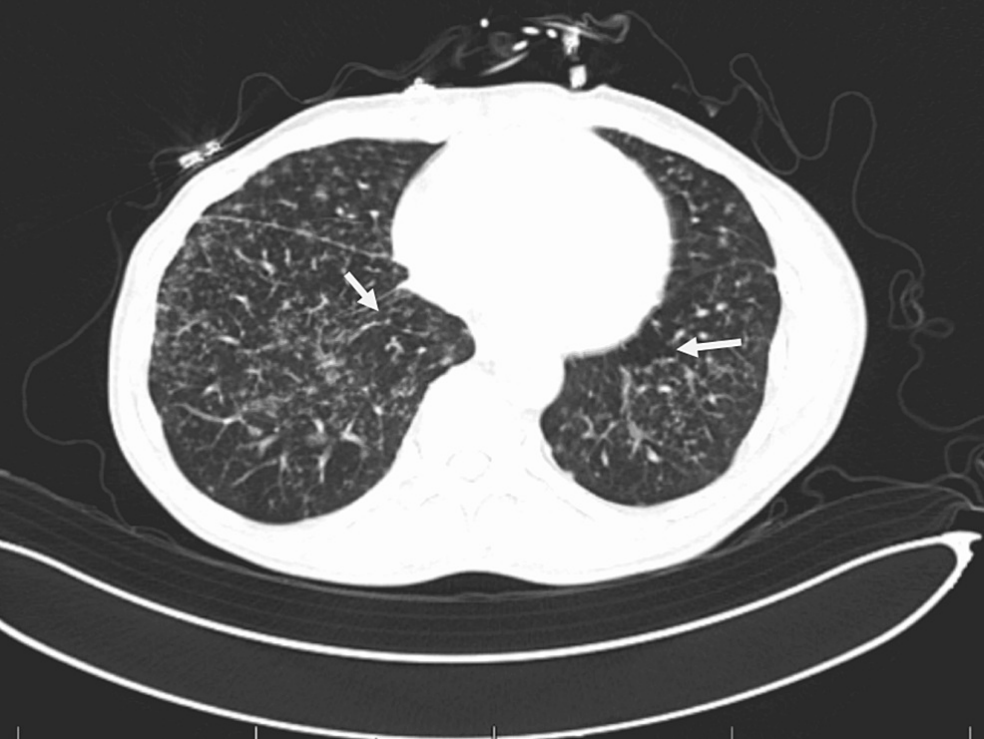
CT of the chest on presentation. White arrows show extensive bilateral reticulonodular opacities throughout the lung parenchyma.
